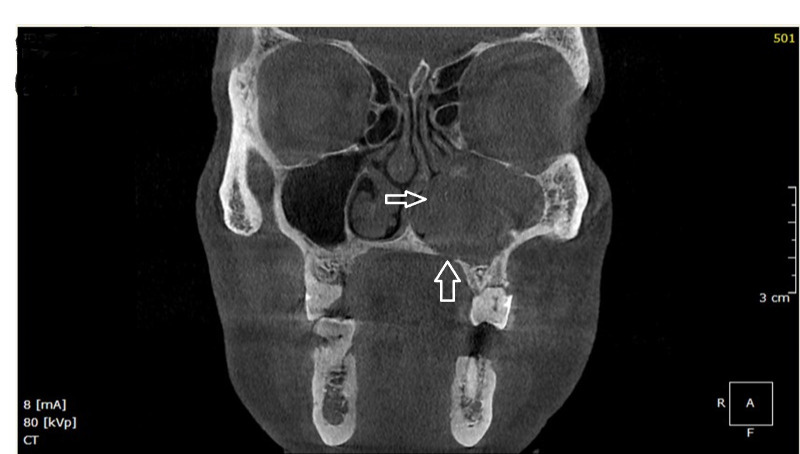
CBCT - Coronal view shows erosion of nasal floor, anterolateral wall and medial wall of maxillary sinus with extension into
